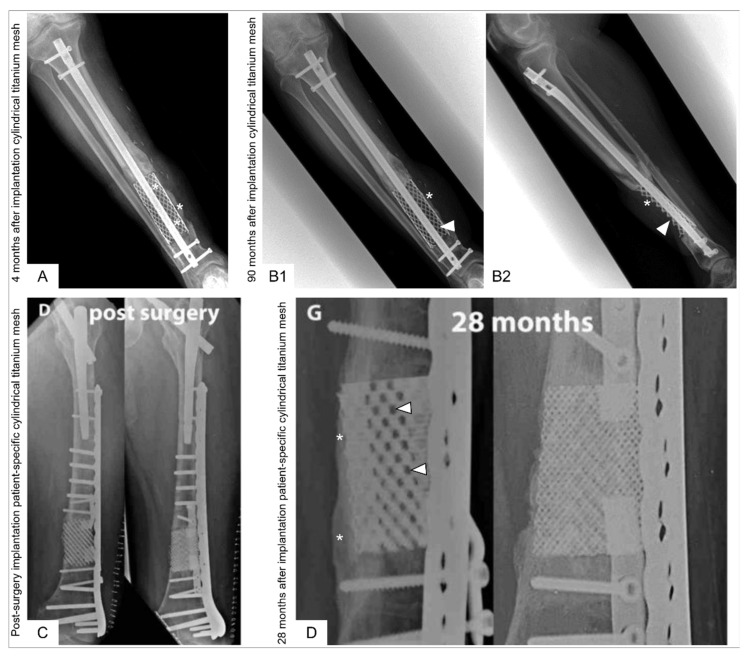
Exemplary challenges and failures in the use of cylindrical titanium mesh cage implants for bone defects. X-ray of a 29-year-old male with a segmental tibial defect and treatment for definitive fixation with an intramedullary nail and cylindrical titanium mesh cage (DePuy Synthes) (A). The asterisks in A1 highlight the graft material four months after grafting, which is packed into and on the outer surface of the cylindrical mesh implant. After 90 months, there is stagnation in the bony consolidation on the surface of the implant (asterisks) compared to plain radiography findings after four months and, in particular, no evidence of a bony structure within the cylindrical titanium mesh cage (triangles) ((B1): anterior–posterior; (B2): lateral). Patient-specific titanium mesh implant (DePuy Synthes) packed with RIA system graft material implanted to treat a femoral defect (C). Twenty-eight months after implantation, a subtle but distinctive callus formed bridging the defect on the implant surface ((D), asterisks indicating bone bridge). However, no bony consolidation was observed in the inner part of the titanium mesh. The triangles in D indicate that the mesh structure is radiolucent, which would not be possible if ABG remodelling and thus successful regeneration of the graft material of the RIA system had occurred. (A,B): reproduced from Supplement of Ref. [126], with permission from the British Editorial Society of Bone & Joint Surgery; (C): reproduced from Supplement of Ref. [129], reprinted with permission from American Association for the Advancement of Science (AAAS).
